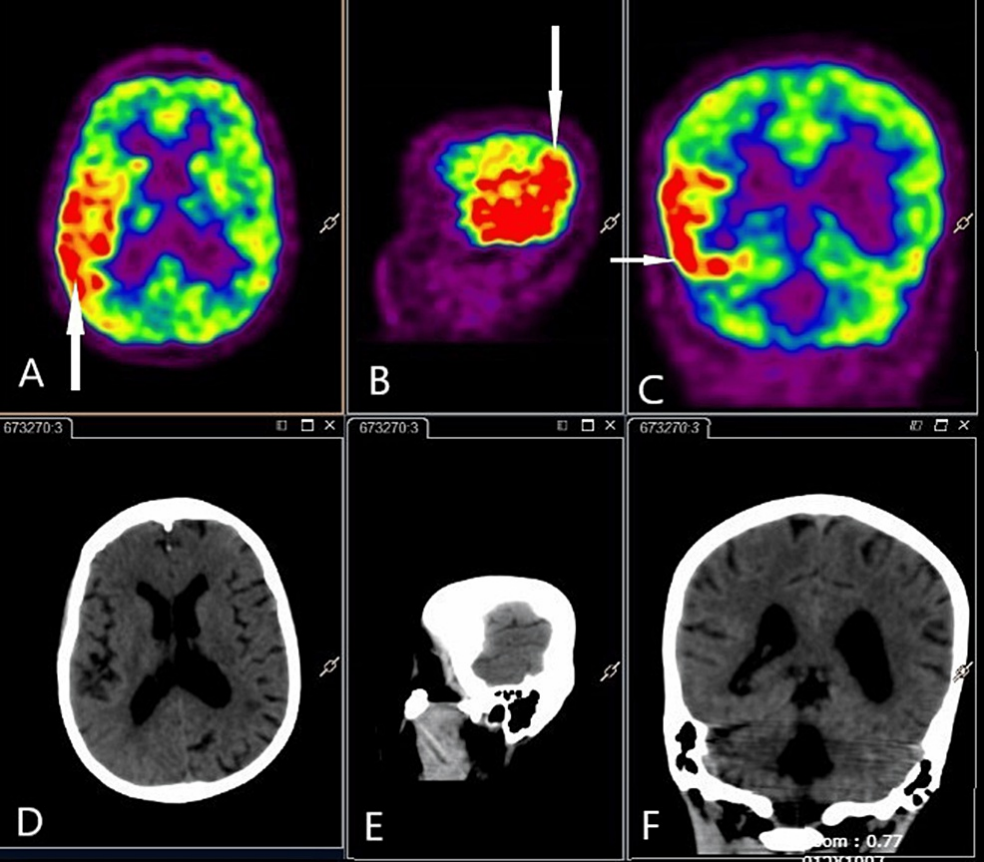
Fludeoxyglucose-PET CT images Fludeoxyglucose-PET CT images (A) axial, (B) sagittal, (C) coronal, sequences showing hypermetabolism, as shown by arrows over the right parieto-occipital region The bottom panel (D, E, F) shows the corresponding plain CT images
